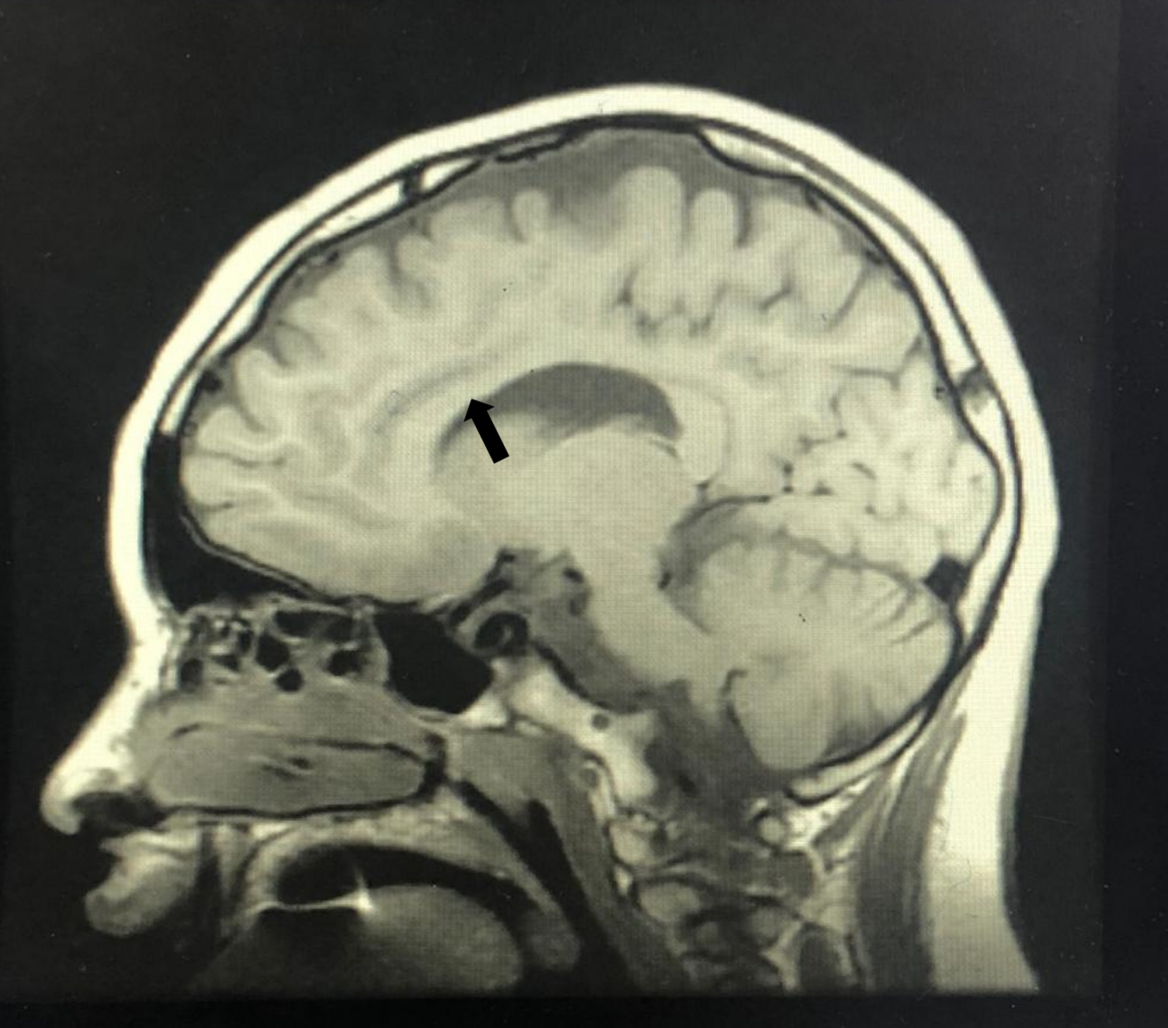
Agenesis corpus callosum; sagittal T1-weighted MRI of the brain shows partial agenesis of the corpus callosum (black arrow)
